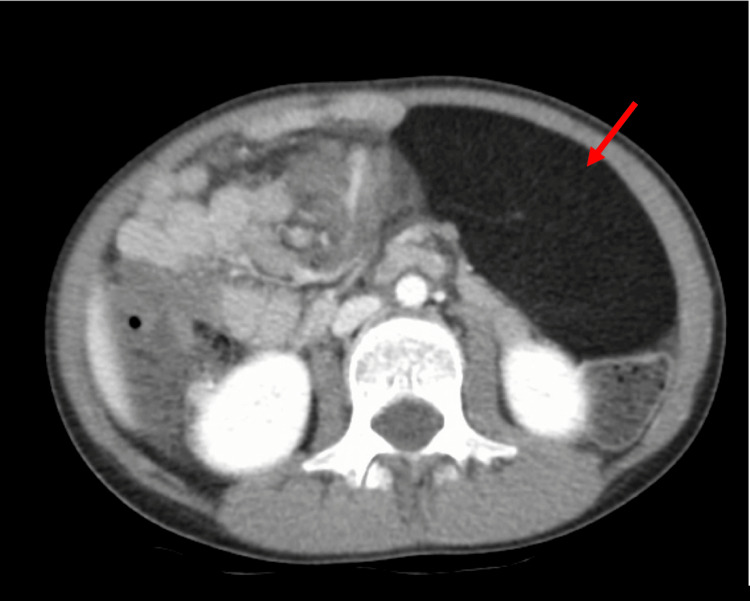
Initial CT scan of the abdomen and pelvis Red arrow: Hypodense omental mass
